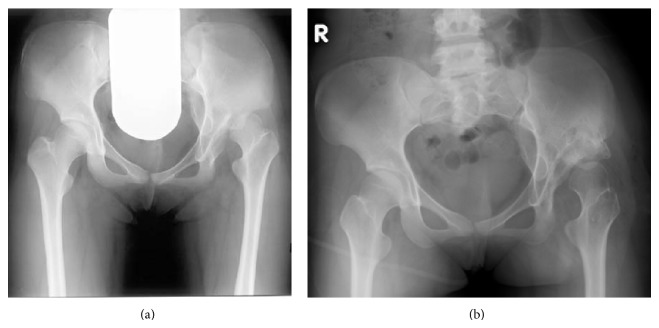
A 13-year-female with left hip subluxation associated with acetabular dysplasia and partial sacral agenesis. (a) A preoperative AP radiograph of the pelvis. (b) An AP radiograph obtained one year after the operation demonstrated partial left sacral agenesis with a rudimentary sacrum had fused with the ilia below the sacroiliac joint.
